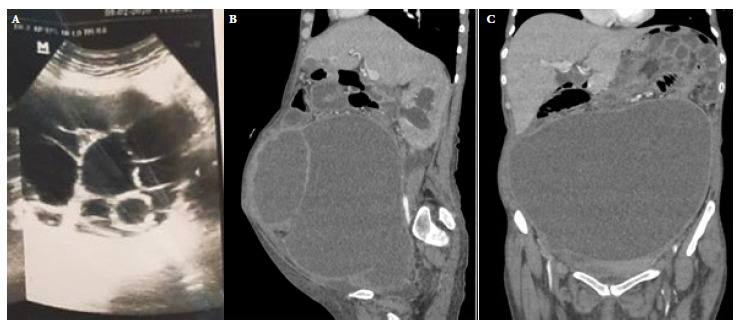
Imaging studies: (A) Ultrasonography performed in 2022: heterogeneous mass, 9 × 10 cm^2^, with multiple cystic images inside, type III according to the Gharbi classification. (B) Sagittal section of multi-slice spiral tomography with contrast. (C) Coronal section of multi-slice spiral tomography with contrast performed in 2022: complex cystic mass of mixed nature, 25 × 19 × 26 cm^3^, with displacement of adjacent intra-abdominal structures and hydroureteronephrosis, type IV according to the Gharbi classification.
